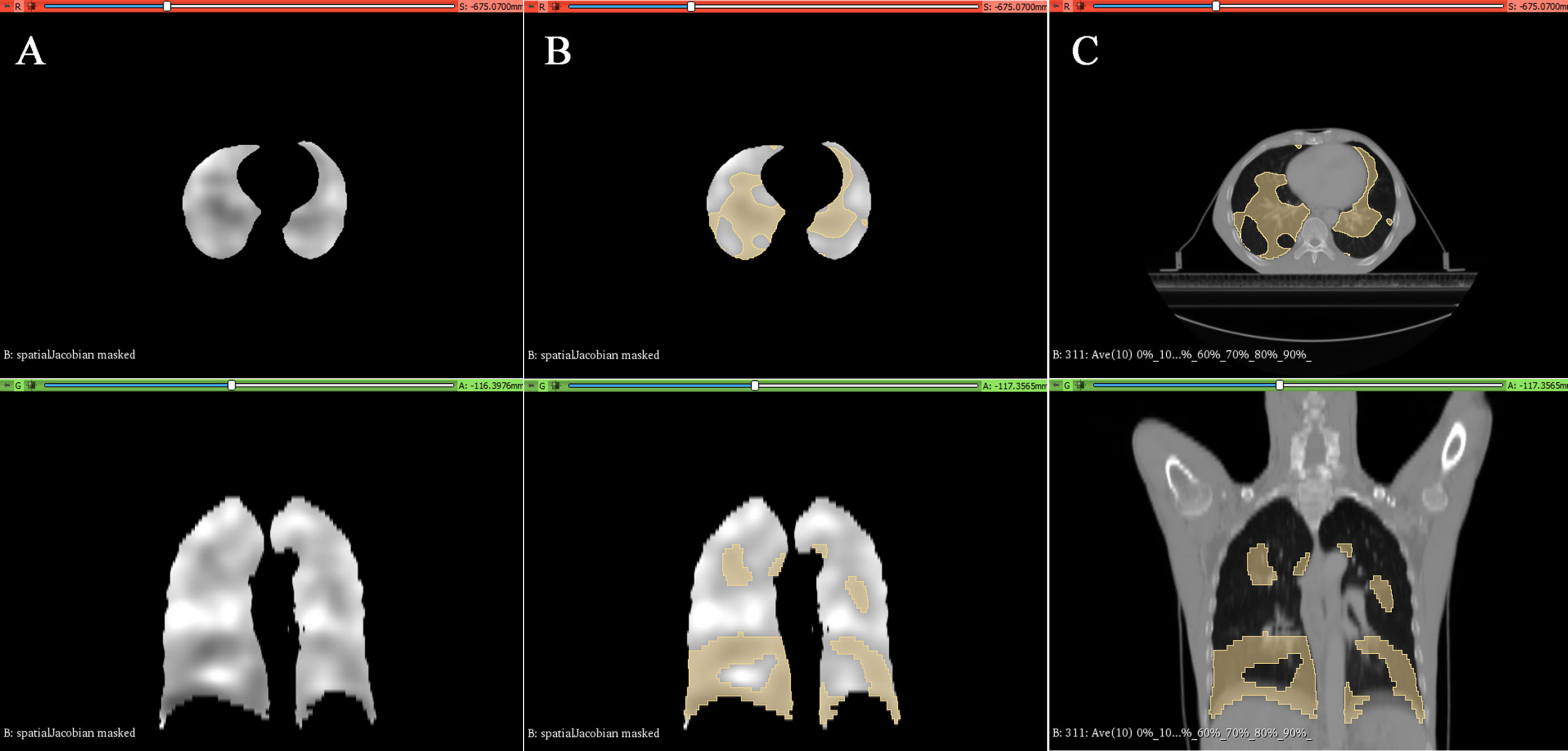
Typical lung ventilation function images generated in 3D-Slicer software. (A) Grayscale image containing the Jacobian value. (B) Defined regions of Jacobian value ≤0.8 (e.g., functional lung). (C) Distribution of functional lungs in the esophageal cancer patient’s anatomical CT.
